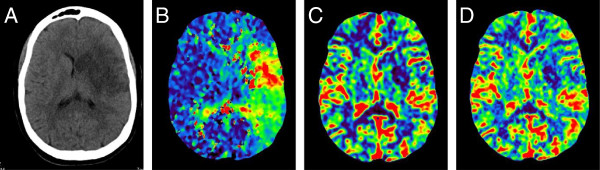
Brain CT imaging post CVA. Brain CT showing completed infarct in left middle cerebral artery territory affecting the left basal ganglia and left insular cortex. Cytotoxic edema present (A). CT perfusion shows increased time to peak (B), reduced cerebral blood volume (C) and reduced cerebral blood flow (D) in the core left MCA territory.
